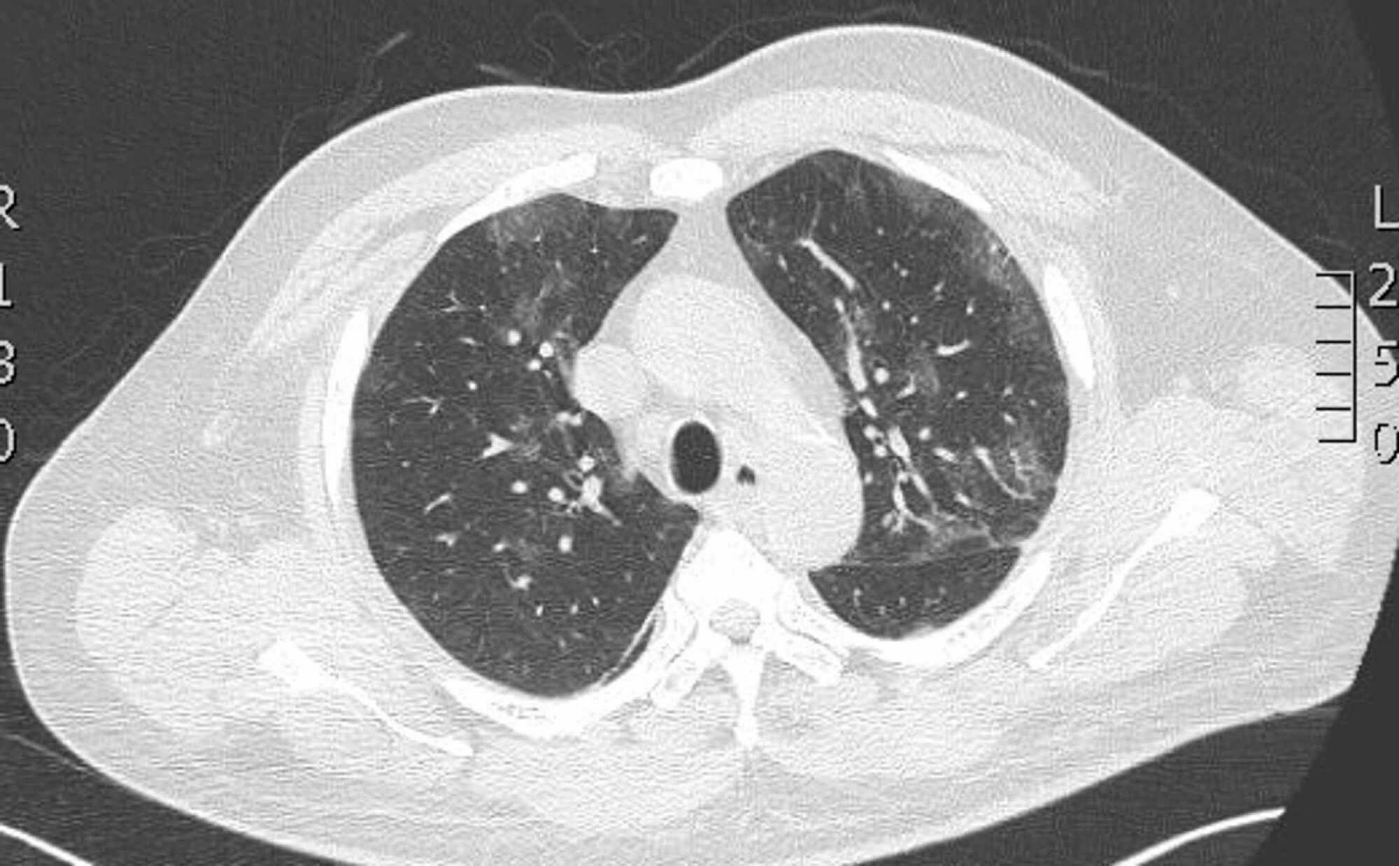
High-resolution CT showing peripheral glass-ground opacities
